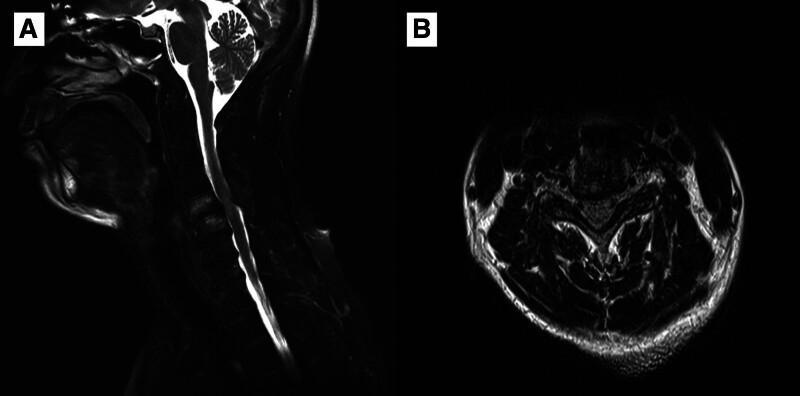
Magnetic resonance imaging in sagittal T2-weighted (A) and axial T2-weighted (B) sequences demonstrates central canal stenosis of C4-C5 due to disc herniation, ligamentum flavum thickening, and intramedullary T2 high signal intensity (evidence of myelopathy).
